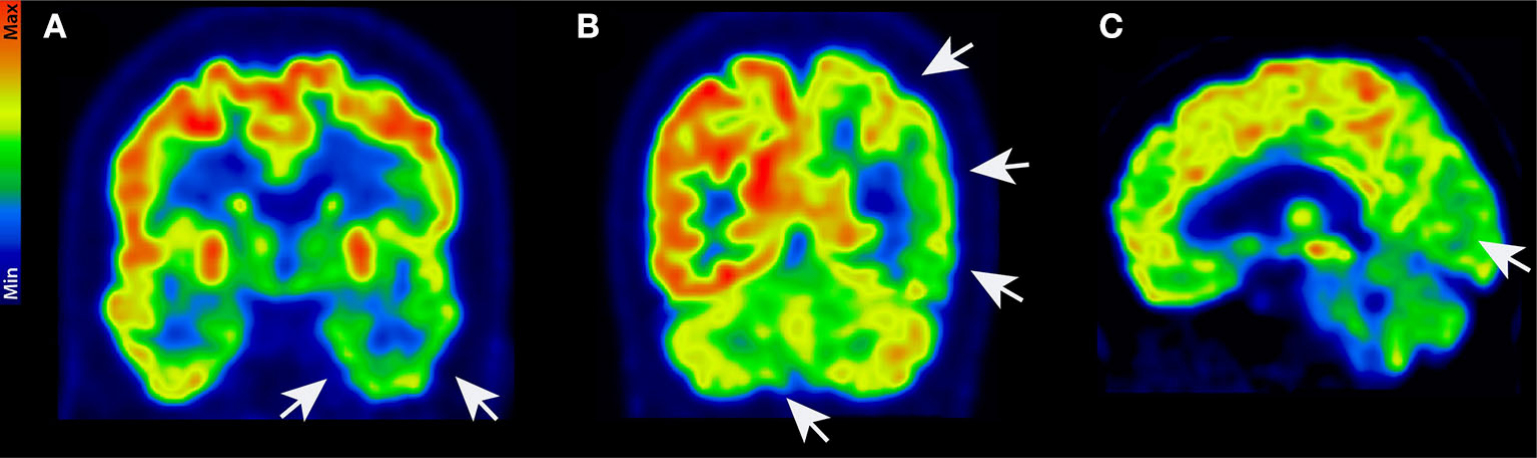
^18^F-FDG-PET. ^18^F-FDG-PET in coronal (A, B) and sagittal (C) view with distinct hypometabolism in the left temporal (A), left parietal, and right cerebellar cortex (B). Furthermore, hypometabolism in the left primary visual cortex was detected (C). White arrows show brain areas with lower tracer uptake.
